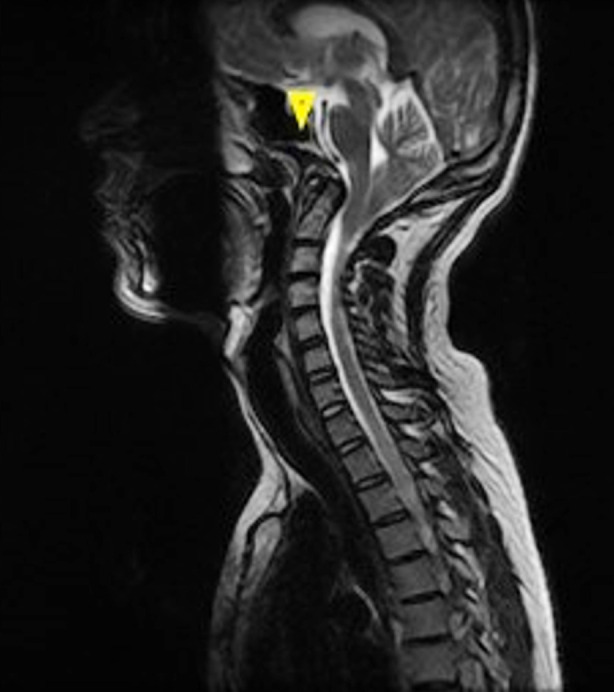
Sagittal T2W sequence of MRI showing clival concavity (yellow arrowhead).
